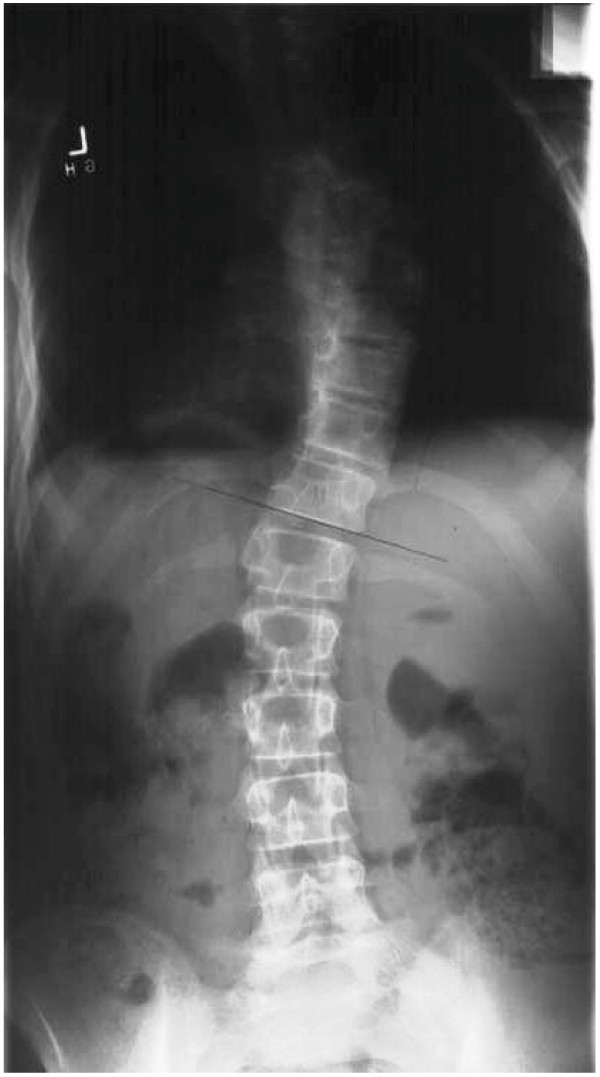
PA plain radiograph Turner syndrome scoliosis.
